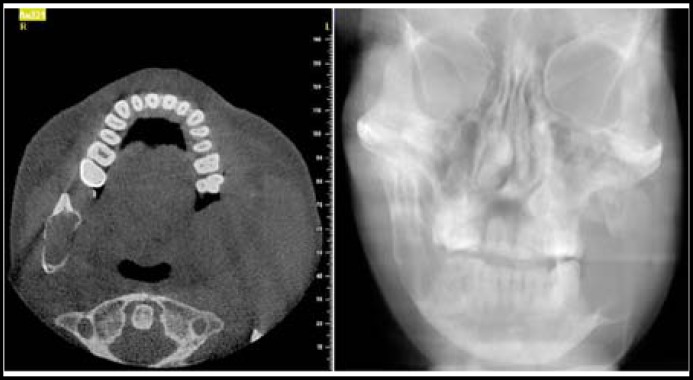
Computed tomography scan of mandible with complete destruction of ramus (Axial view and posteroanterior view).
